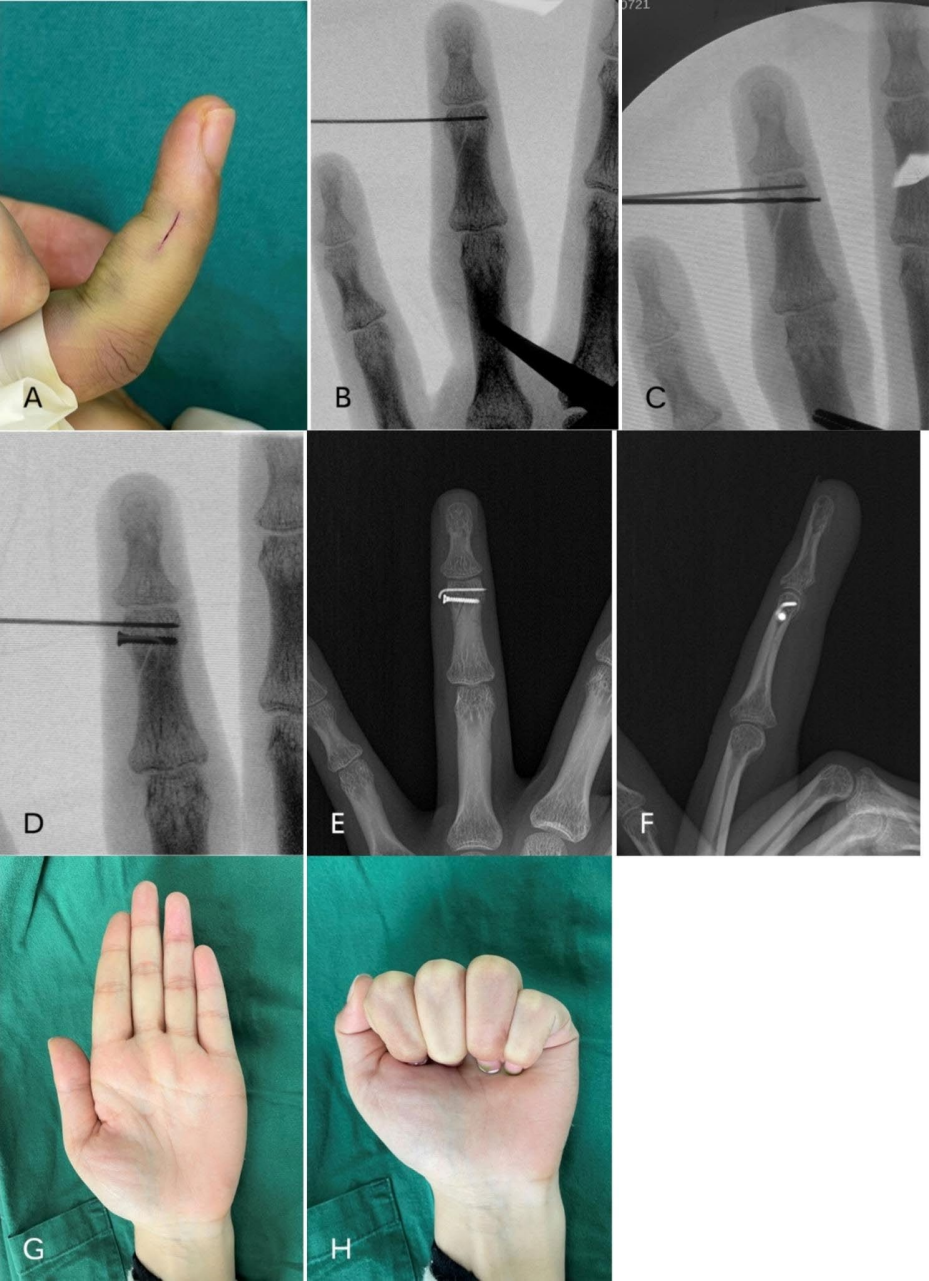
A 27-year-old female patient with an AO type B3.1 fracture of the middle phalanx of the left ring finger received K-wire combined with screw. a Micro incision on the side of finger fracture. b to d showed the operative process of K-wire combined with screw. e, f were the postoperative radiograph. g, h showed the functional outcome at 2 months
